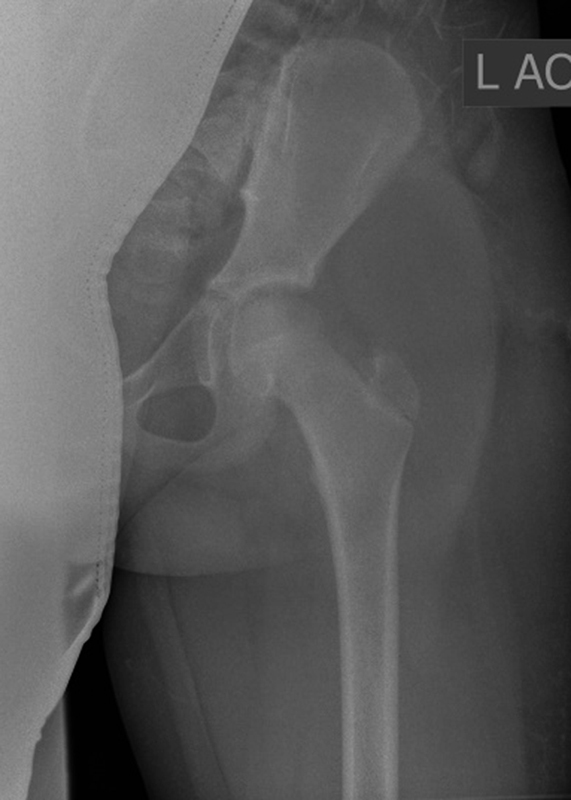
Anteroposterior (AP) radiograph shows the concentric reduction of the hip after arthroscopic treatment.
